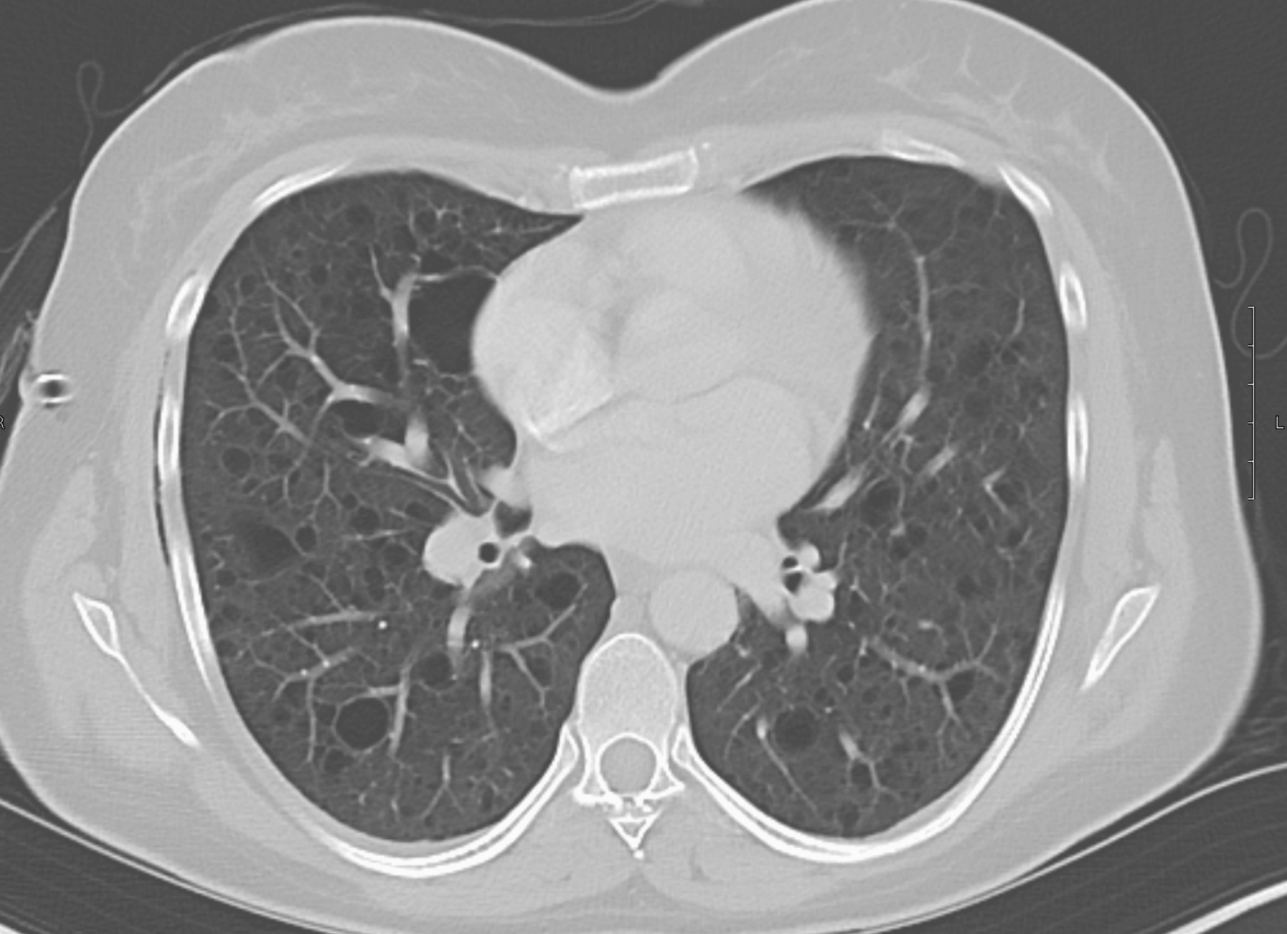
Contrast-enhanced CT of the middle lung zones showing numerous bilateral discrete cystic spaces with very thin or imperceptible walls Lung volumes are normal with no evidence of emphysema CT: computed tomography
